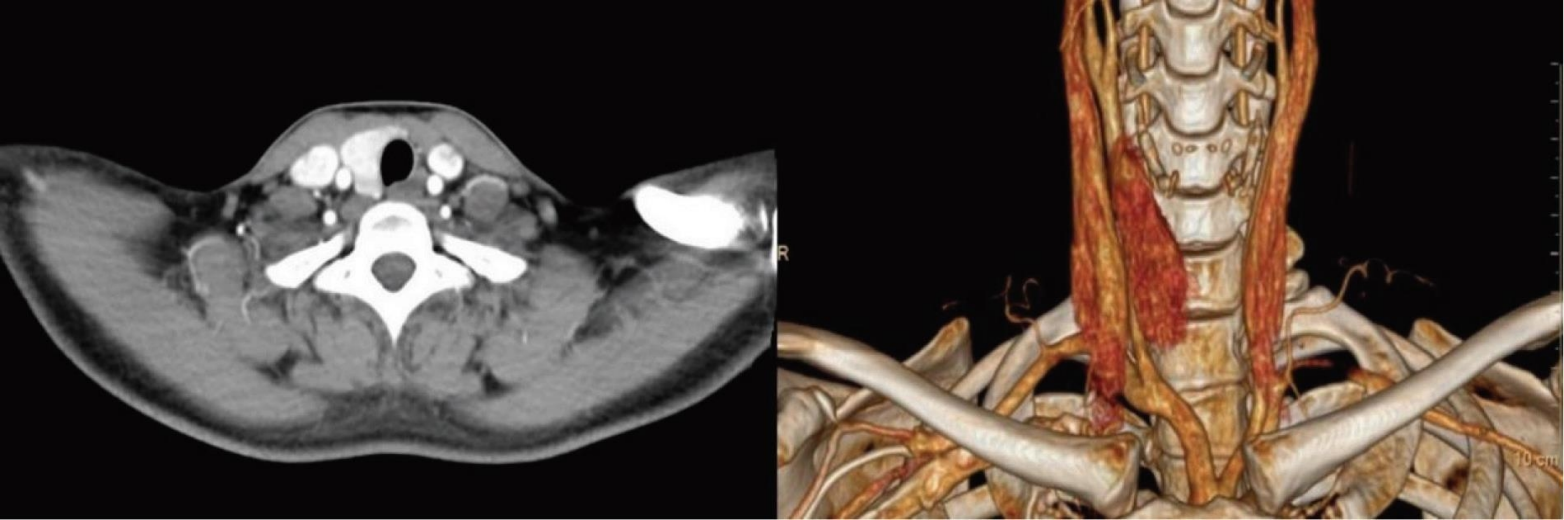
CT and 3D reconstruction of the neck.
